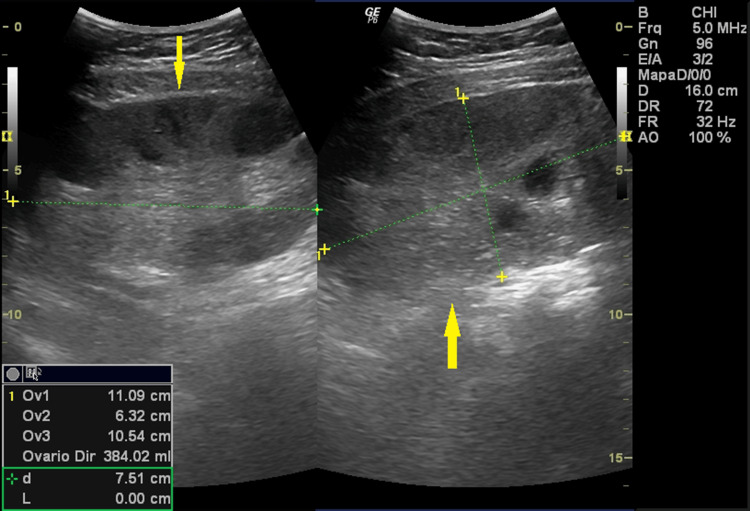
Right ovary with adnexal torsion. Transvaginal ultrasound with amplitude Doppler demonstrating an enlarged right ovary with heterogeneous parenchyma and absent vascular flow. The arrow indicates the ovary exhibiting sonographic features of torsion.
